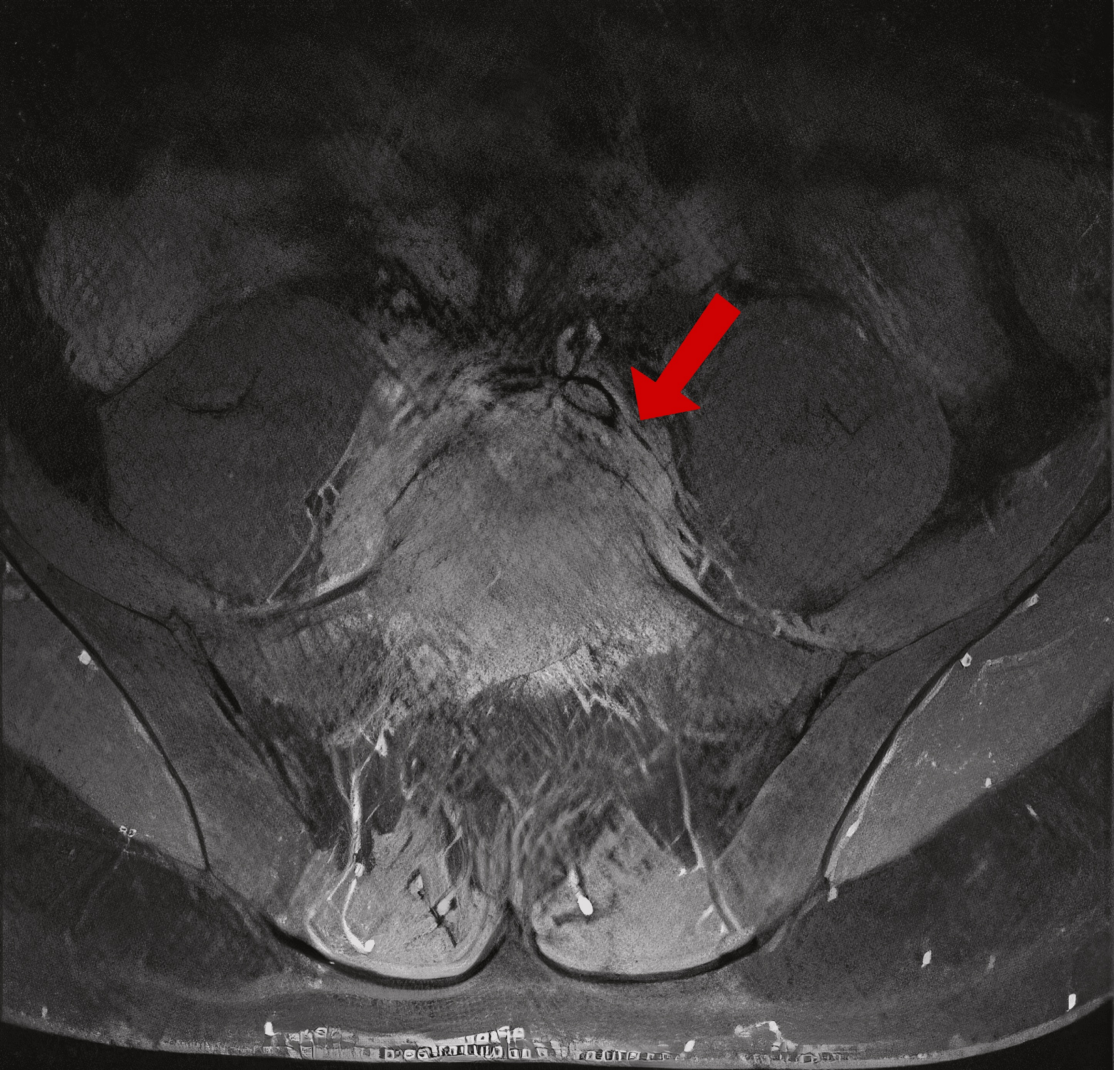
MRI lumbar spine, December 2, 2024 MRI: magnetic resonance imaging Axial T2-weighted MRI at the L4-L5 level showing circumferential epidural and paravertebral soft-tissue thickening (arrow)
